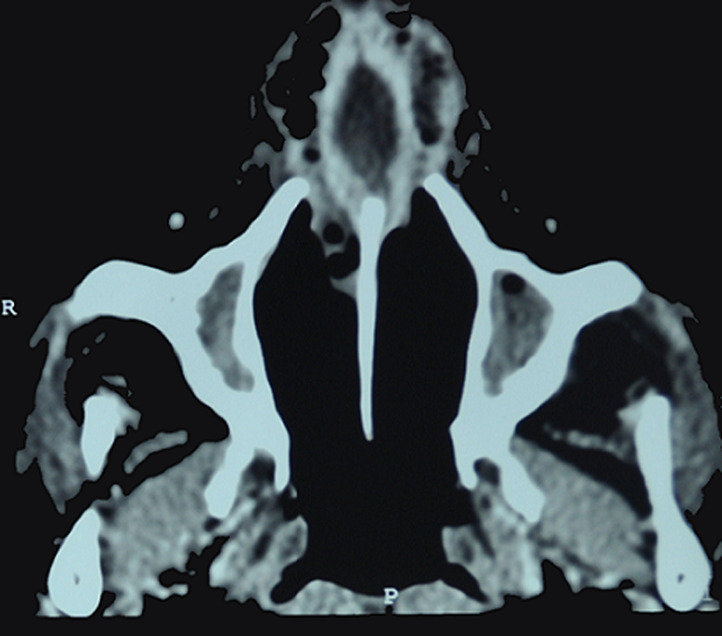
an axial computed tomography scan shows a collection in the anterior nasal septum
